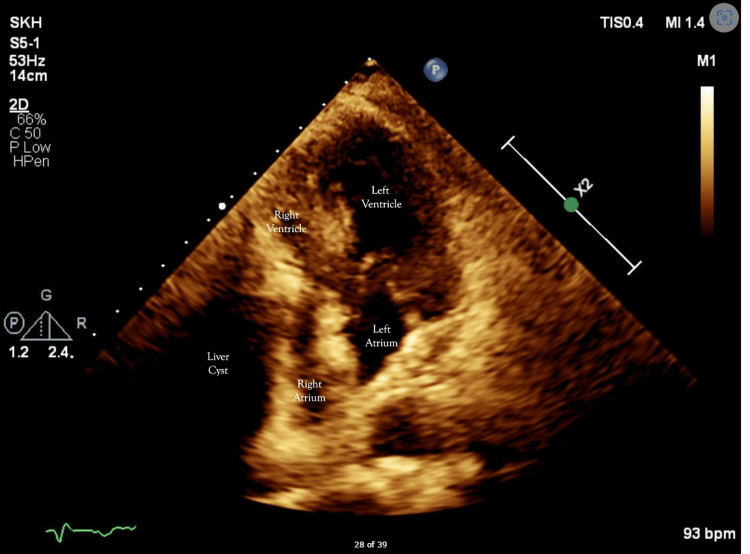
Transthoracic echocardiogram demonstrating compression of the right heart by the adjacent large liver cyst (four-chamber view)
